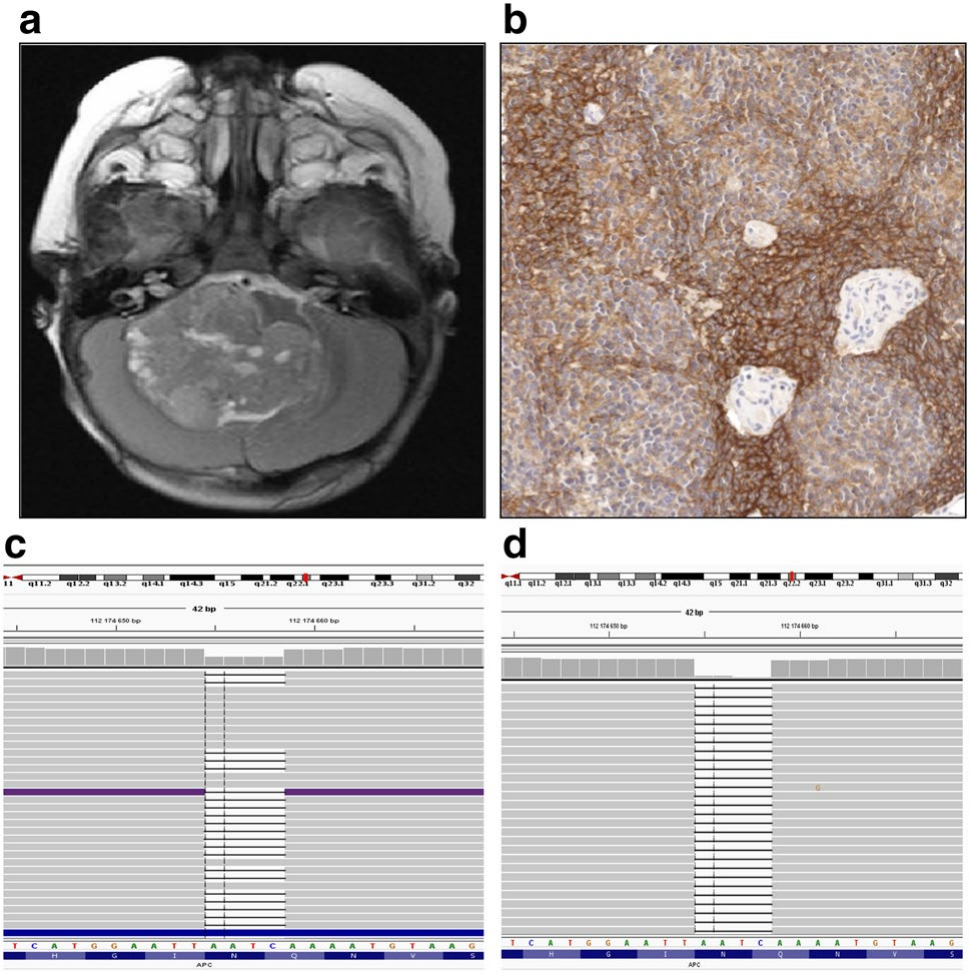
Medulloblastoma tumor with *APC* mutation. Magnetic resonance image of the tumor with cerebellar midline location (a); ALK immunopositive reaction present in > 80% of tumor cells (b); result of NGS analysis displaying the presence of the c.3366_3369del *APC* variant in heterozygous state in DNA extracted from patient’s leukocytes (c) and in homozygous state in tumor sample (d). Preparation was scanned at original magnification × 40 and digital magnification presented on image is × 20
